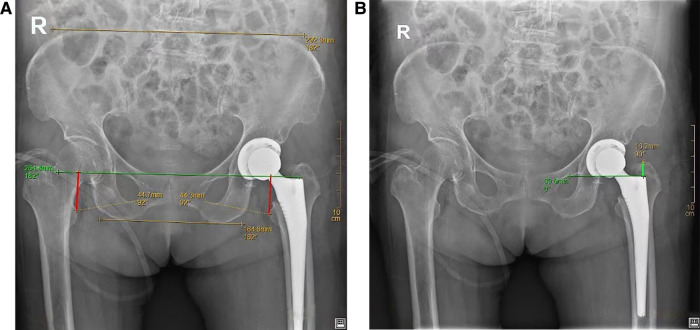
(A) Postoperative x-ray measurement of the bilateral leg length discrepancy using the preoperative method (red line); (B) postoperative imaging of the ipsilateral apex–shoulder distance (green line).
